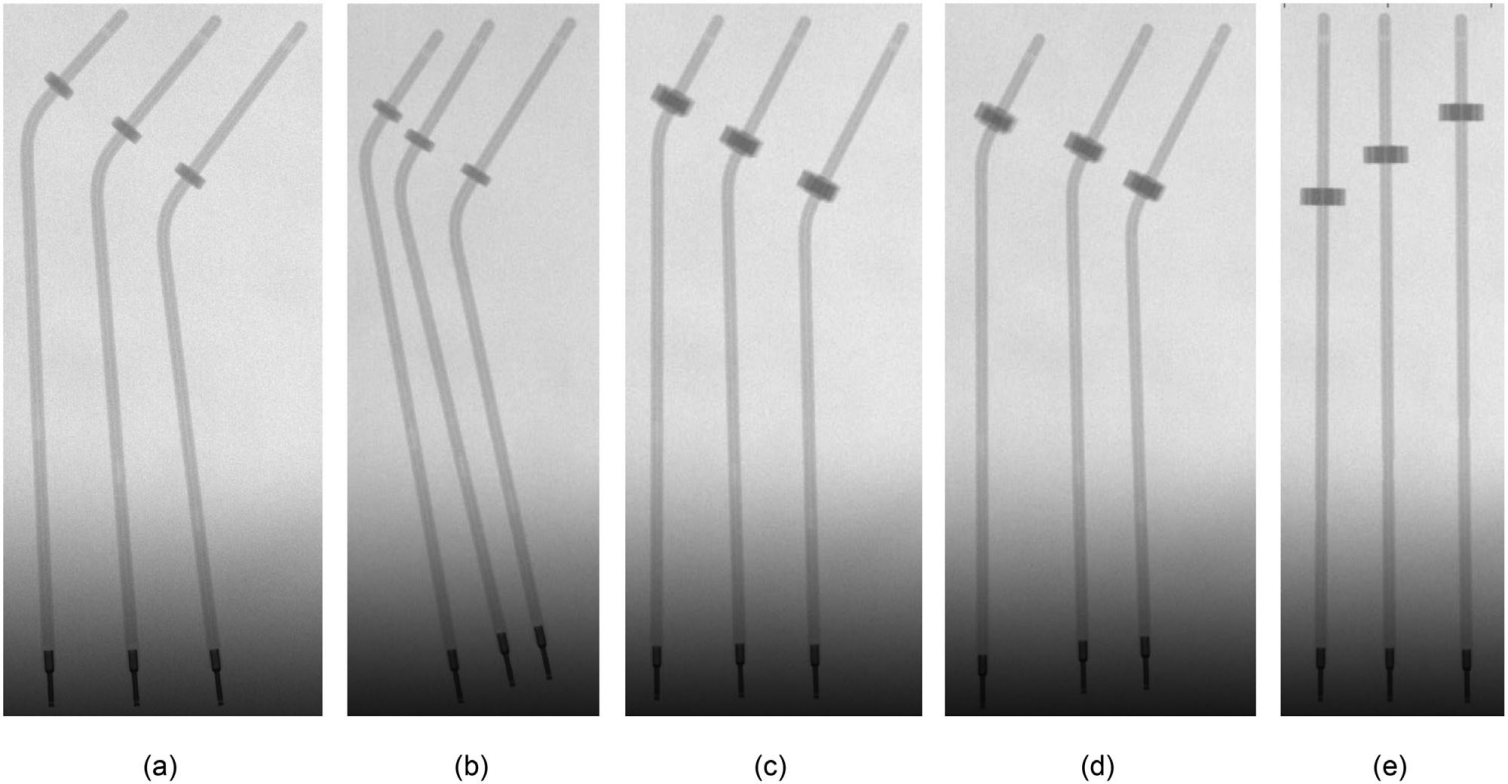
Radiographs of the tandem applicators, with no marker wires in place. Applicators are arranged from left to right, 40 mm, 60 mm, and 80 mm. Shown in (a) are the Set A 45° tandems, (b) the Set B 45° tandems, (c) the Set A 60° tandems, (d) the Set B 60° tandems, and (e) the 90° tandems.
